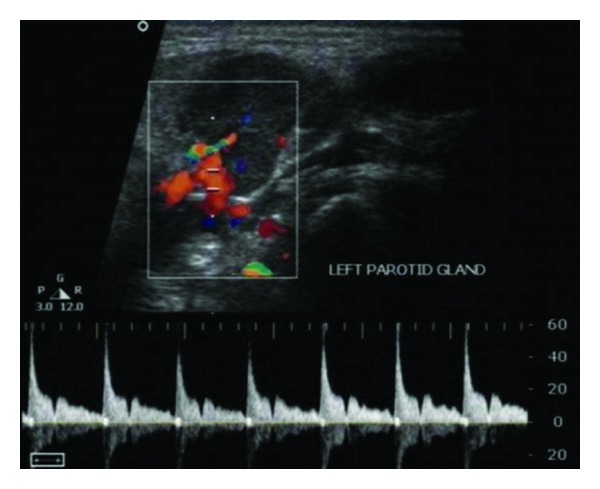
Colour doppler spectral waveform within the intralesional flow shows arterial type of flow with relatively high resistance.
